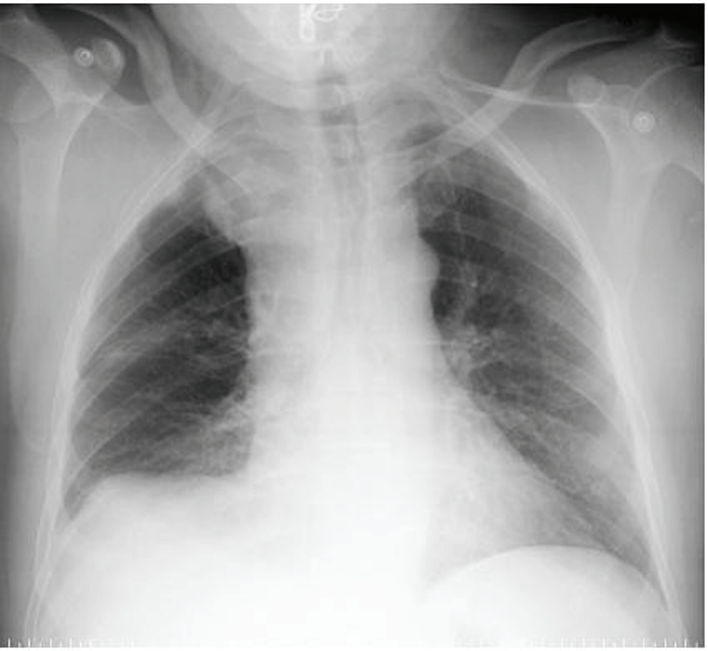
Chest radiograph in posteroanterior projection is shown in which a pleural effusion in the right lower field and a consolidation image at the top ipsilateral chest and adjacent to the mediastinum can be observed.
